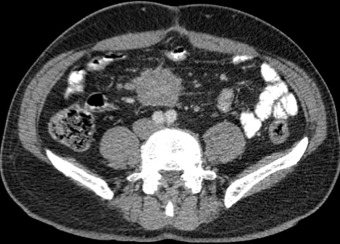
Axial CT scan with contrast 12 months after the diagnosis, showing stability of the lesion
